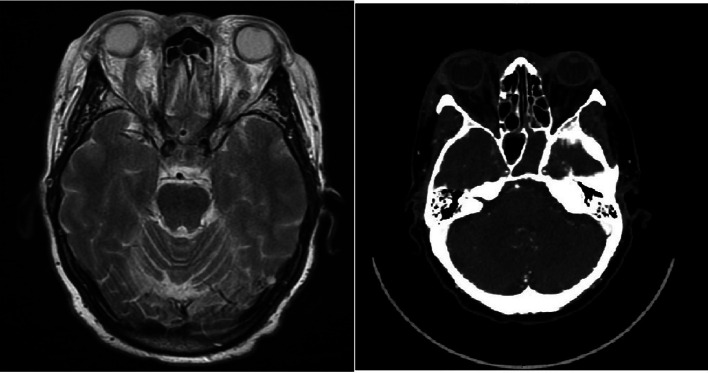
Imaging MRI and CT pre-treatment
